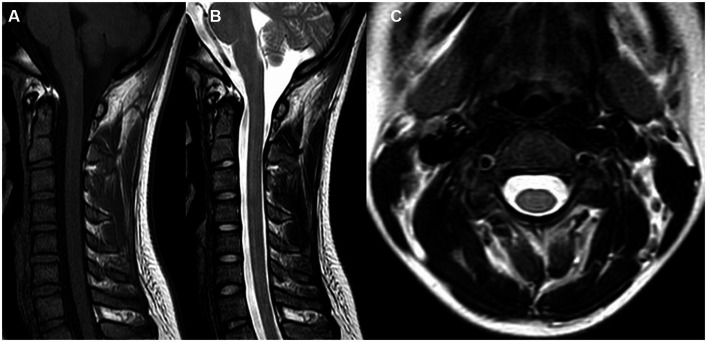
MRI images of the cervical spinal cord (A–C) were obtained. These included sagittal T1-weighted (A), T2-weighted (B), and axial T1-weighted (C) images. Notably, no lesions were observed in the cervical spinal cord.
